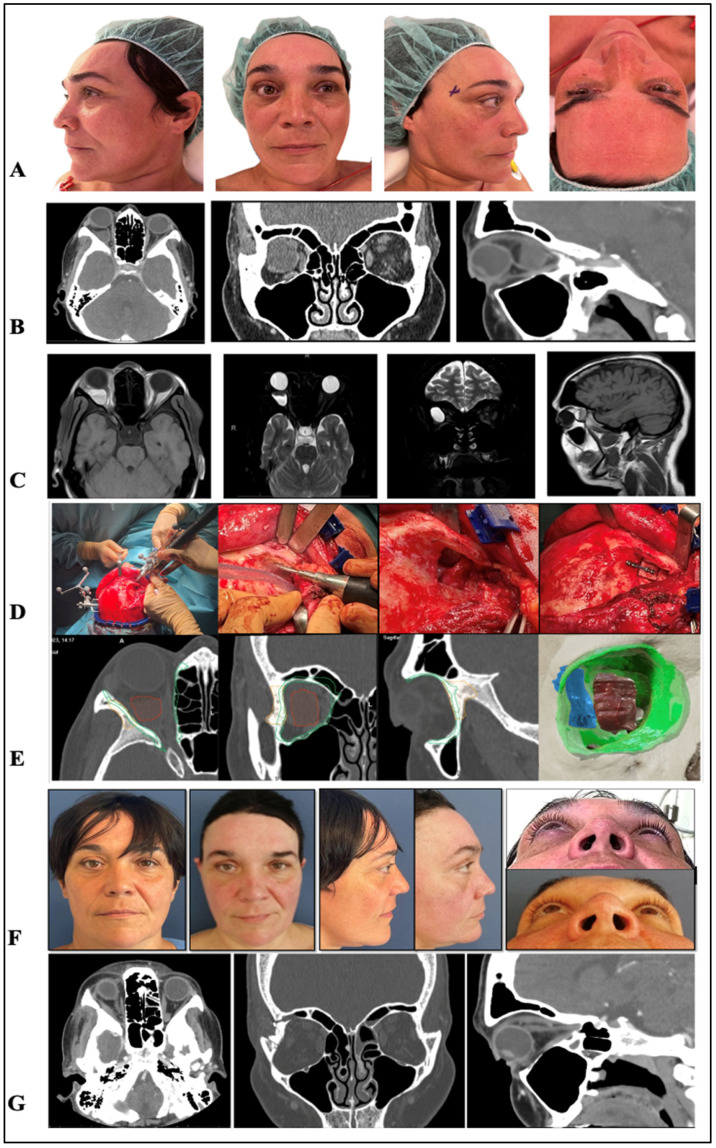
(A) Preoperative clinical images showing asymmetry due to right-side proptosis. (B,C) CT and MRI scans demonstrating a cystic lesion with fluid–fluid level in the superotemporal and retroconal right orbit, particularly evident on T2 sequence. (D) Sequential intraoperative views (left to right): navigation sensors placed on the skull and piezoelectric handpiece; planned right lateral orbitotomy guided by navigation; lateral access to the lesion after orbitotomy; and repositioning of the lateral wall with a miniplate and screws. The approach used was an extended hemi-coronal technique, followed by complete cyst resection by Ophthalmology. (E) Virtual planning: orbital walls (green), tumor (red), and lateral wall segment for orbitotomy (blue), with axial, coronal, and sagittal CT slices. (F) Postoperative clinical images showing correction of asymmetry. (G) CT scan at 2 months demonstrating correct repositioning of the lateral wall, restored intraorbital relationships, and correction of exophthalmos, with preserved ocular motility and pupillary reflex despite mild postoperative inflammation.
